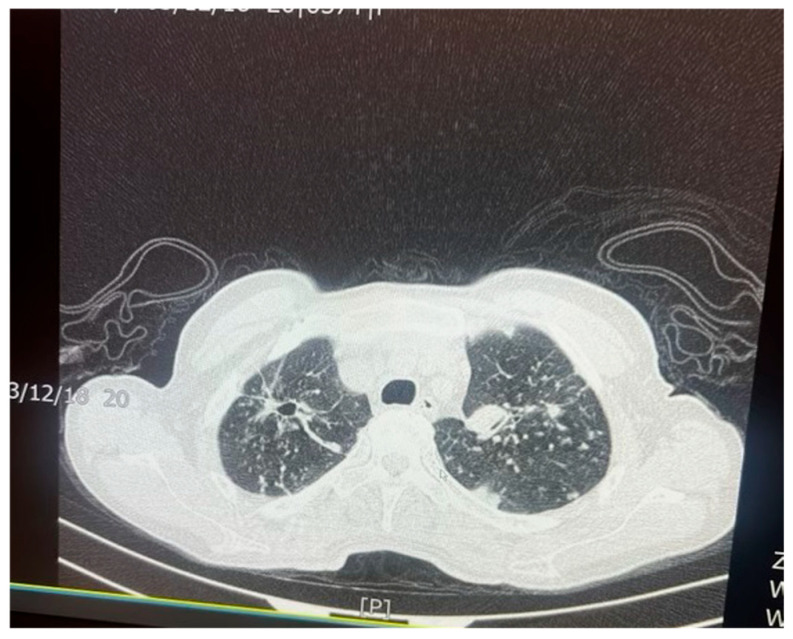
An axial chest CT scan of a 57-year-old female patient with pulmonary TB, showing bilateral pulmonary cavities and multiple nodules. The cavities are seen predominantly in the upper lobes, with irregular thickened walls, suggestive of active TB. The nodular lesions surrounding the cavities represent granulomatous inflammation, which is characteristic of TB infection. These findings are typical of post-primary TB in adults.
